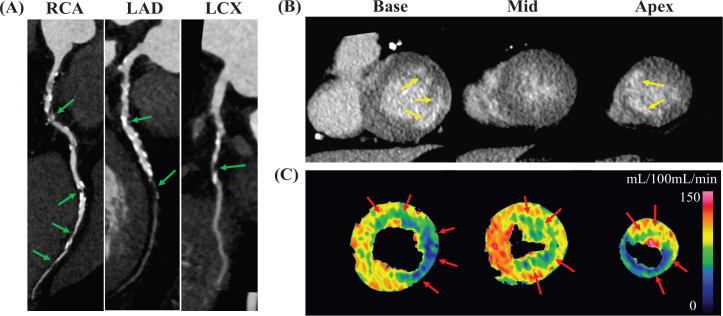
Representative case of CAD assessment by comprehensive cardiac CT. The figure shows results of CAD assessment in a 70-year-old male with esophageal cancer using comprehensive cardiac CT, including (A) CCTA images (curved multiplanar reconstruction), (B) LECT images (short axis), and (C) dynamic CTP maps (short axis). On the CCTA images, significant stenoses (green arrows) in all three coronary arteries were observed. The LECT images revealed enhancement areas showing infarction (yellow arrows) in the basal lateral and apical septal walls. The dynamic CTP maps demonstrated perfusion abnormalities suggestive of myocardial ischemia in myocardial areas that did not display infarction. CAD: coronary artery disease; CT: computed tomography; CCTA: coronary computed tomography angiography; RCA: right coronary artery; LAD: left anterior descending artery; LCX: left circumflex artery; LECT: late enhancement computed tomography; CTP: computed tomography perfusion.
